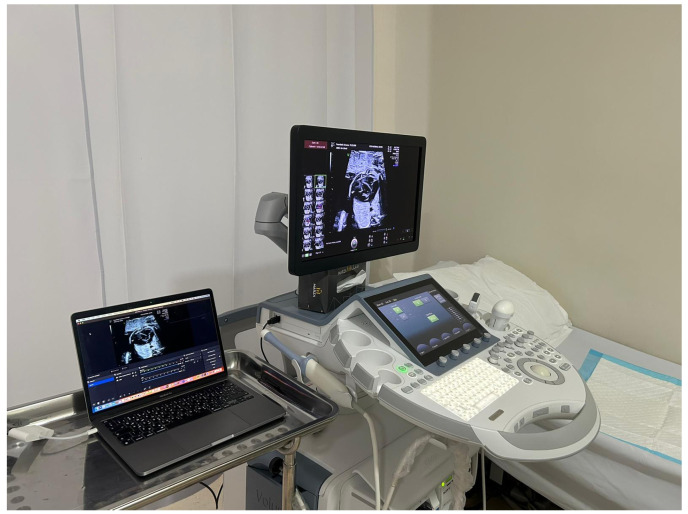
Set-up for imaging and tele-counselling. In this picture, the paediatric cardiologist has been replaced with an ultrasound image.
